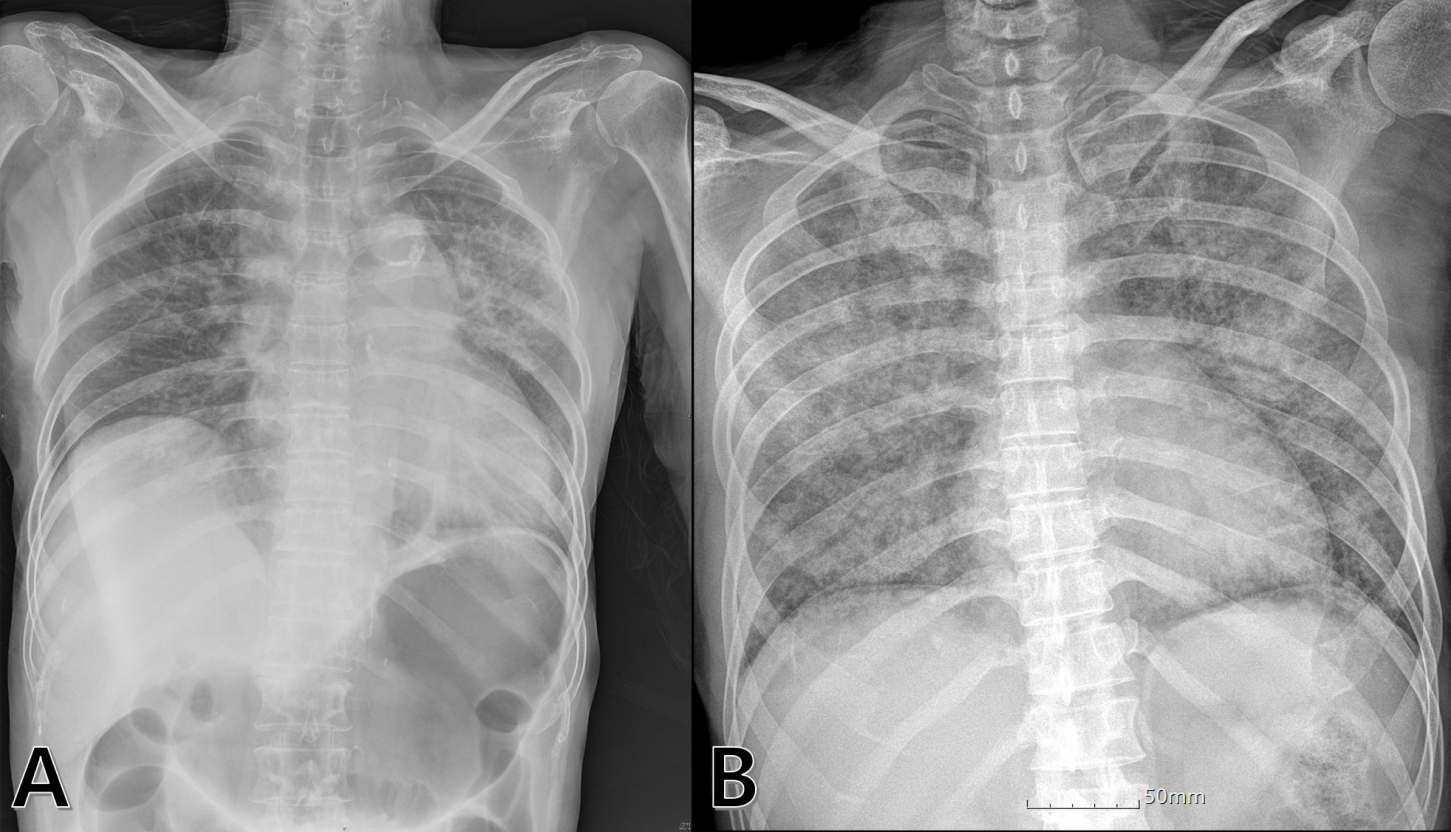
An example of a plain chest radiograph with (A) and without (B)
